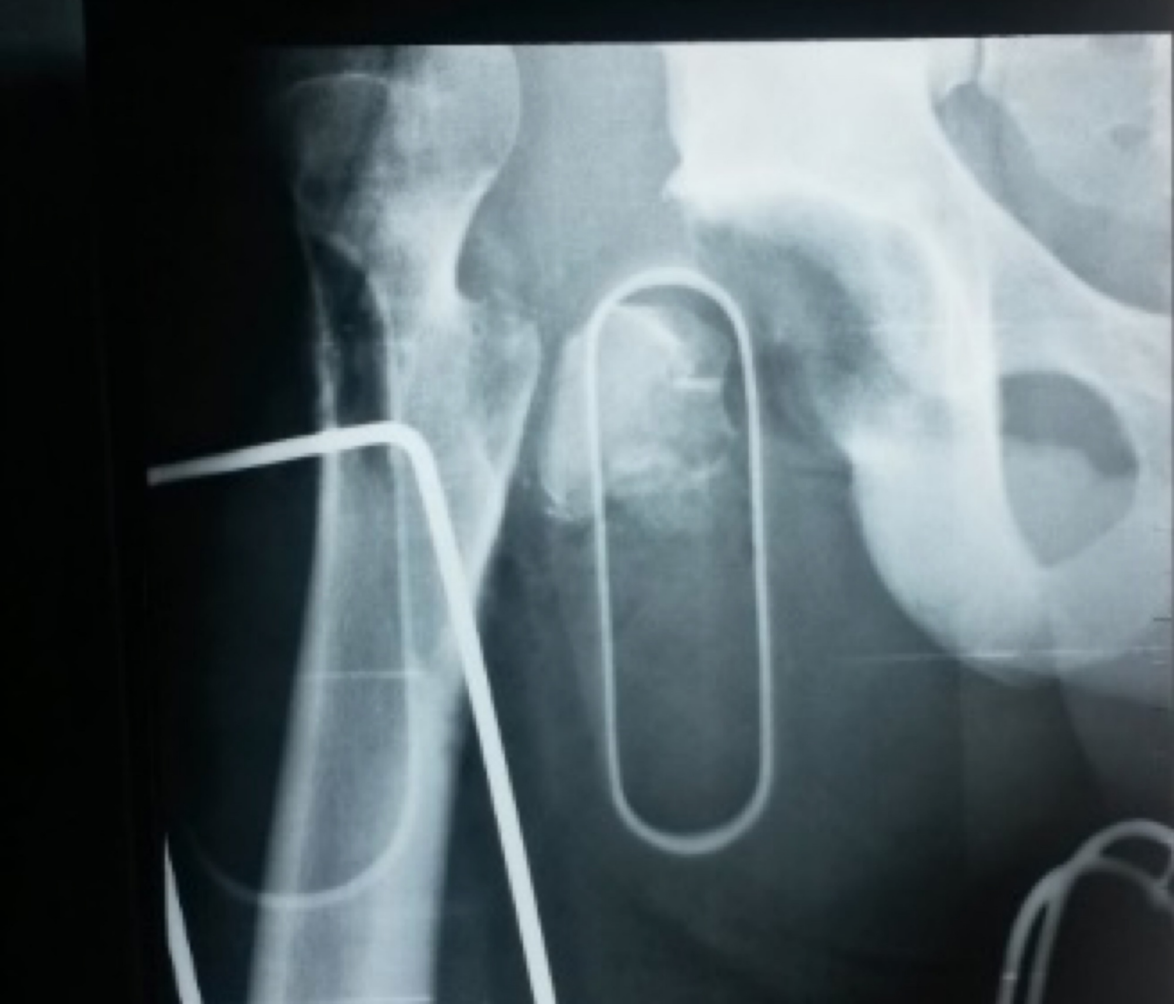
A radiograph of the right hip showing the dislocation with a fracture of the greater trochanter
